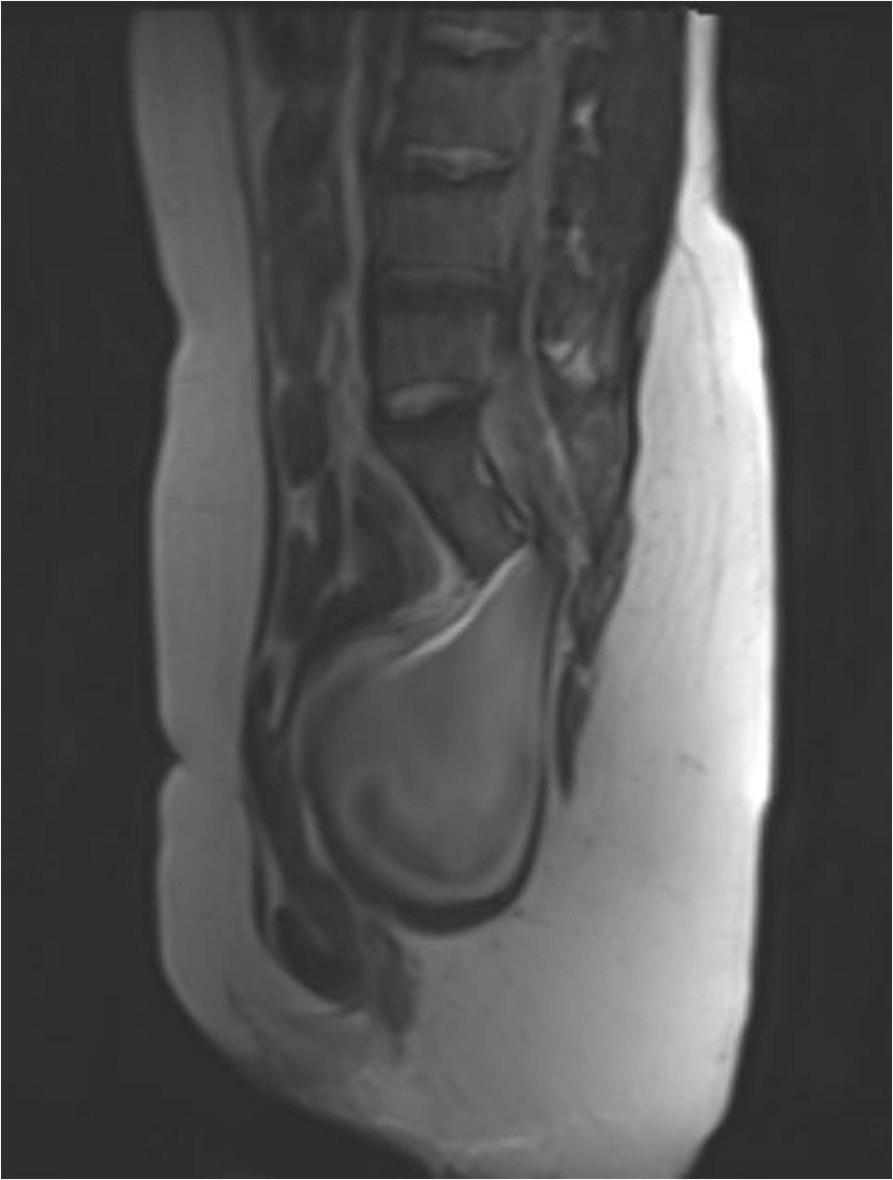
Preoperative MRI showed: agenetic sacrum (from S4 and S5) with an anterior meningocele was seen.
